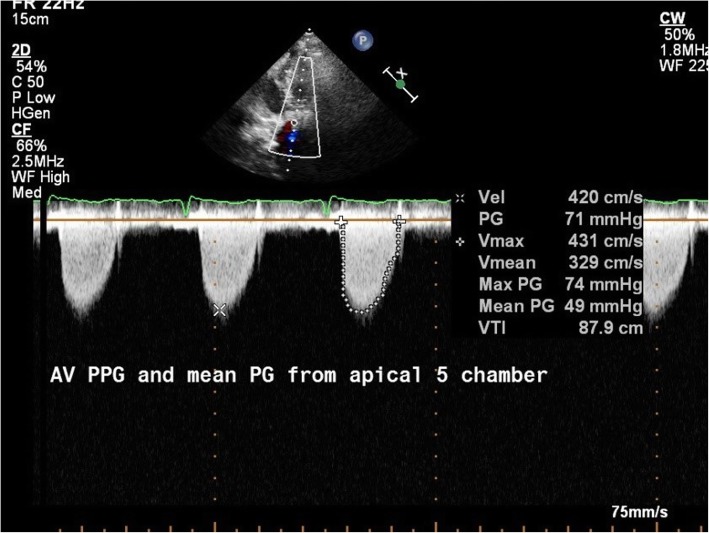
Continuous wave Doppler use in measurement of MPG and PPG across the aortic valve in patient no
